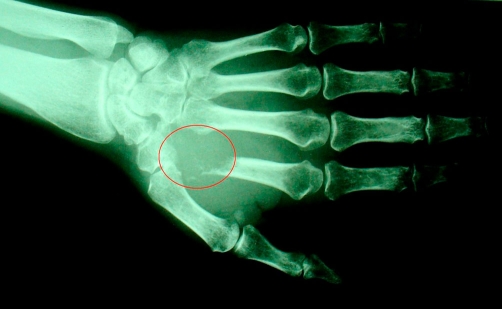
X-Ray imaging of the left hand showing osteolytic lesion of the second metacarpal bone.
